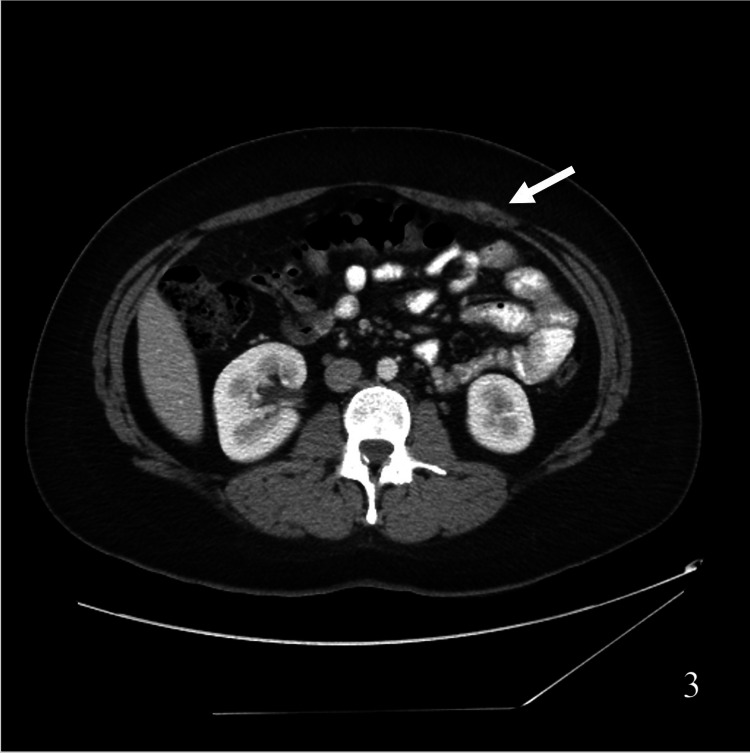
Axial abdominal CT scan demonstrating evidence of very early (three months) port site metastases after cholecystectomy.
